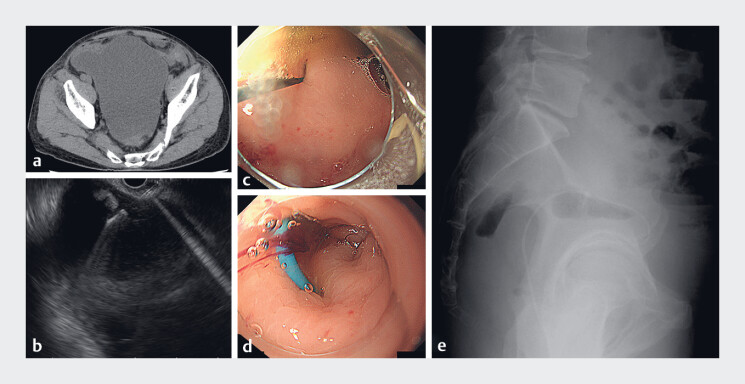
Initial endoscopic ultrasound (EUS)-guided pelvic abscess drainage.
